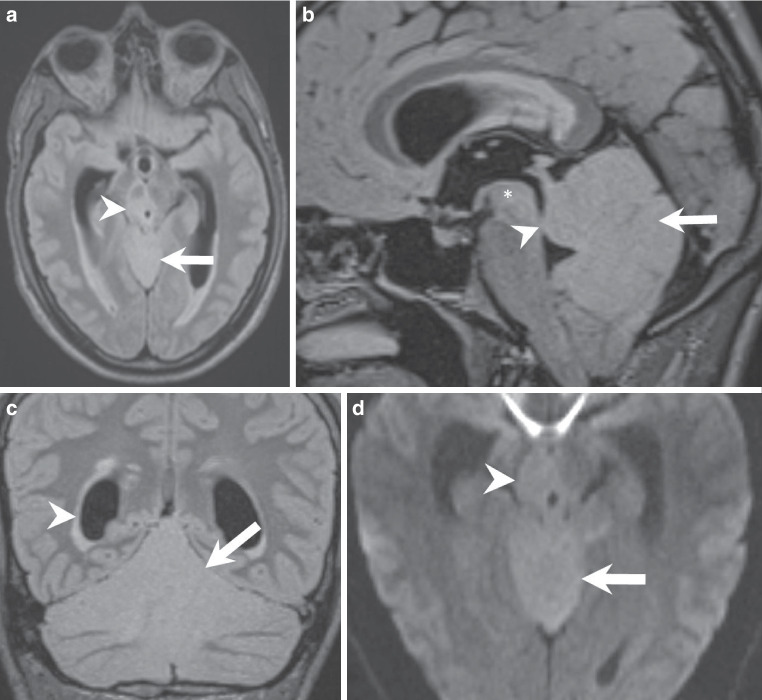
MRI findings on admission. a–c Axial (a), sagittal (b), and coronal (c) fluid-attenuated inversion recovery (*FLAIR*) images demonstrate a homogeneously hyperintense, space-occupying lesion involving the cerebellar vermis as well as the anterior and posterior cerebellar lobes (arrows), with extension into the mesencephalon (a, arrowhead; b, asterisk). The aqueduct seems to be obrcucted by the mas lesion (b, arrowhead) with subsequent dilatation of the lateral ventricles (c, arrowhead). d Diffusion-weighted imaging (*DWI*) shows marked restriction of diffusivity within the lesion at the level of the vermis (arrow) and the mesencephalon (arrow head), suggestive of hypercellularity
